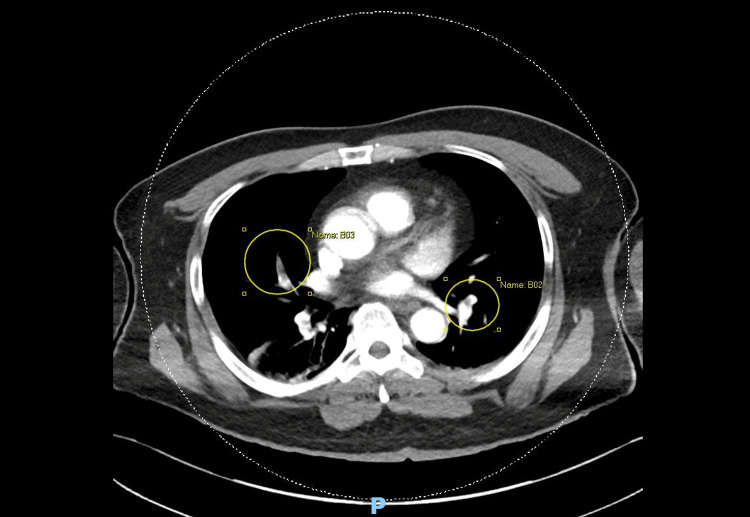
Computed tomography pulmonary angiography (CTPA). Filling defects were noted around the subsegmental branches of the pulmonary arteries in the middle lobes of the lungs (yellow circles).
